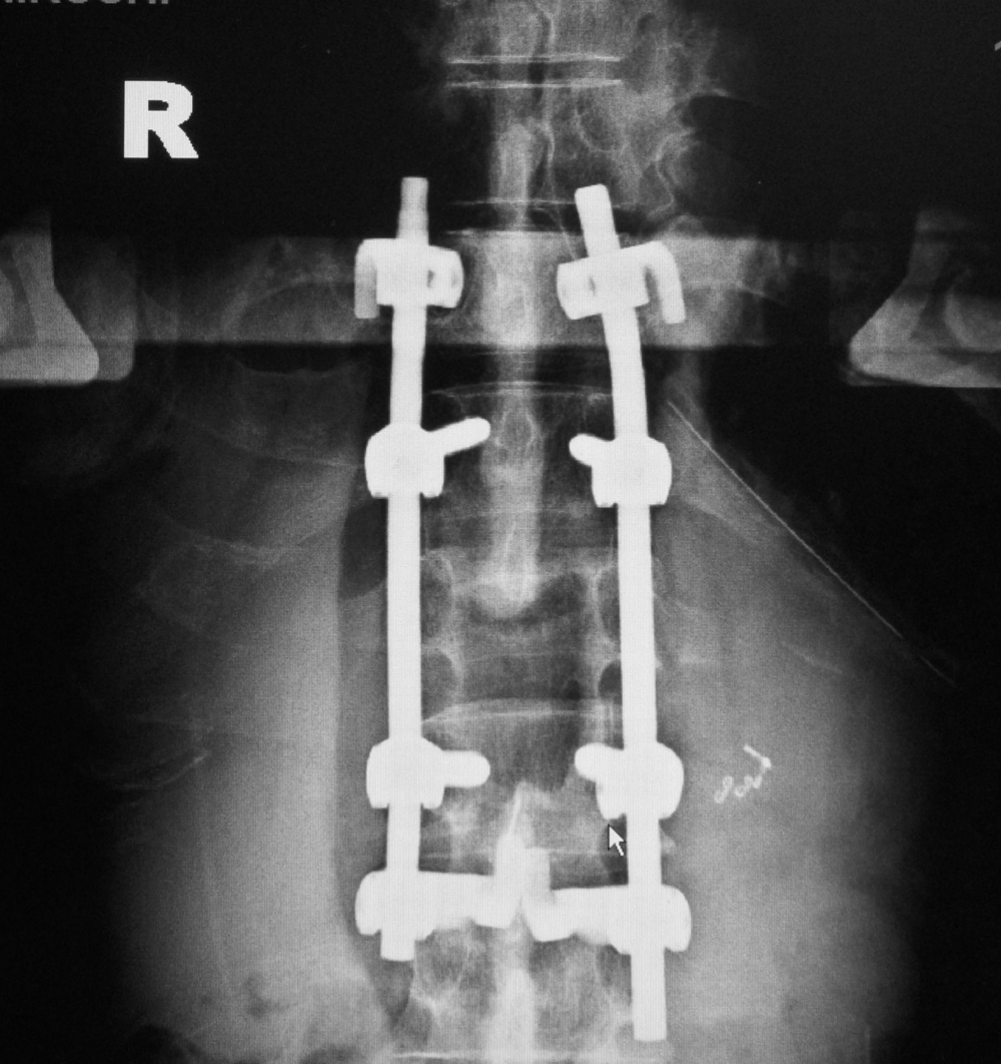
Postoperative roentgenogram.
